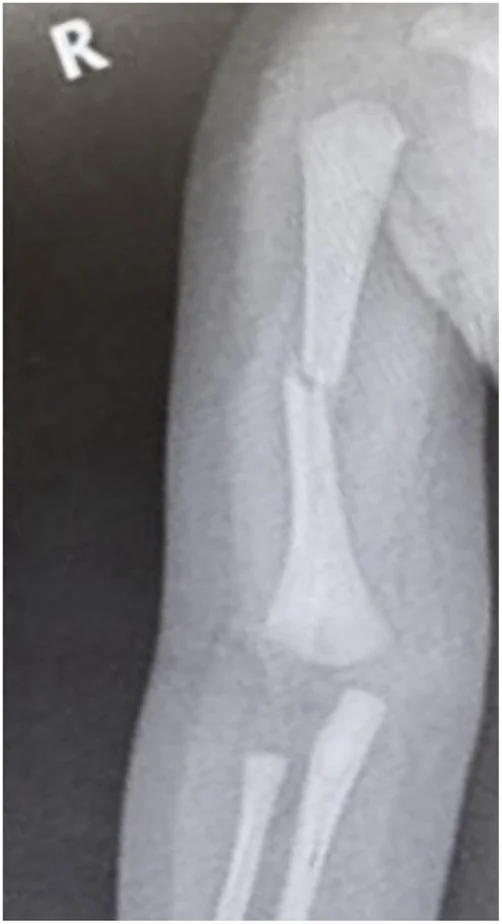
Plain radiograph demonstrating a minimally displaced midshaft fracture of the right humerus in the neonate.
